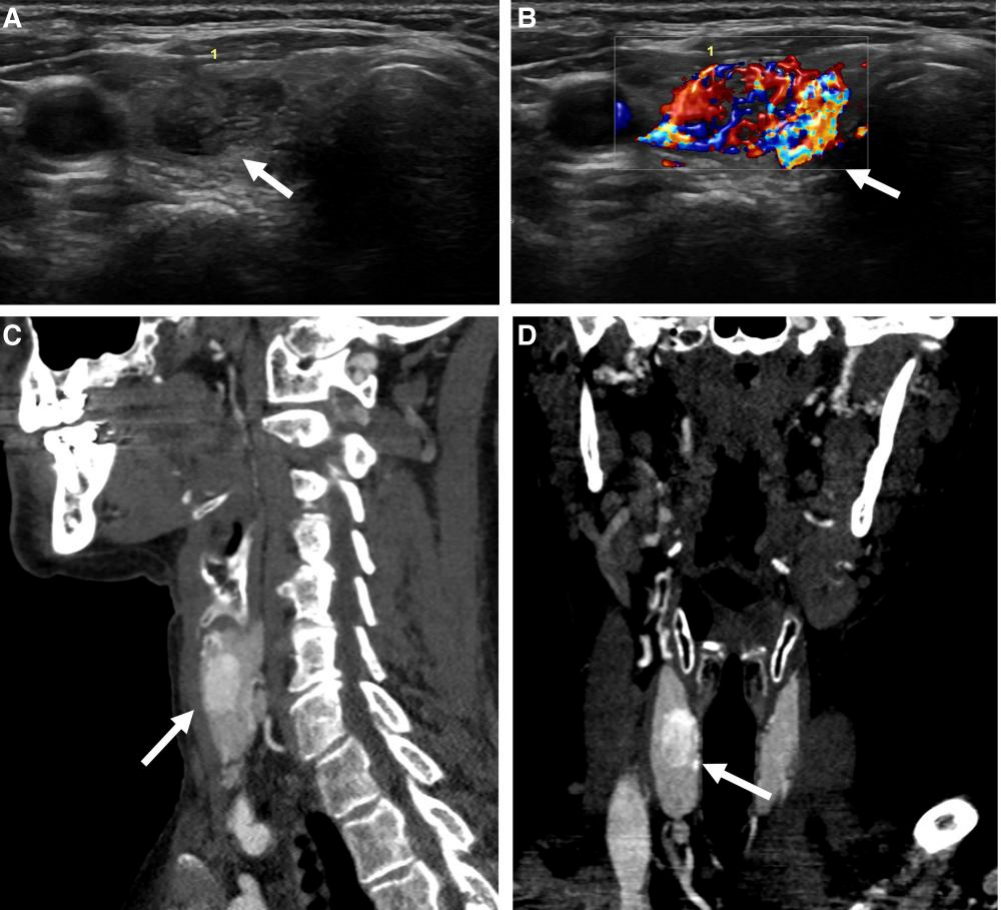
Thyroid paraganglioma images. A and B: Thyroid ultrasound revealed a 1.7 × 0.9 × 1.2 cm right solid, hypoechoic, hypervascular, wider than tall thyroid nodule with lobulated margins and without evidence of extrathyroidal extension. C and D thyroid paraganglioma in the right thyroid lobe on computed tomography (CT) neck with contrast.
